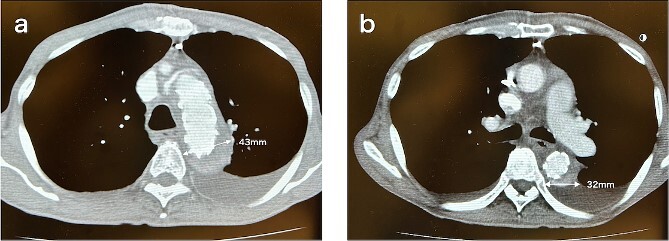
CT 5 days after the initial surgery revealed no endoleak and good remodeling of the descending aorta at the levels of the aortic arch (a) and the carina of the trachea (b).
